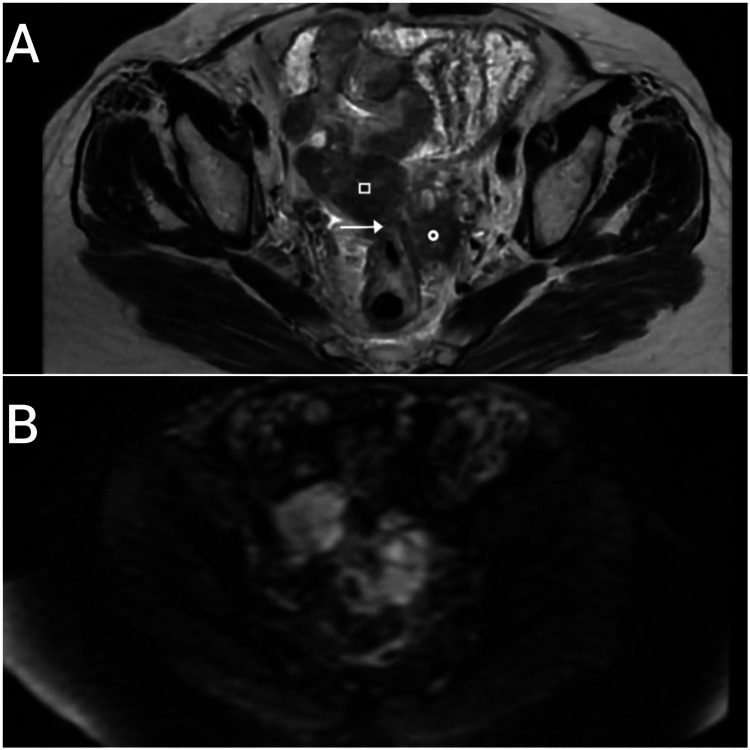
Axial T2 and diffusion of pelvic magnetic resonance imaging (MRI) (A) Axial T2 pelvic MRI: rectosigmoidien invasion (arrow), mass in the right ovary (square), mass in the left ovary (circle). (B) Diffusion pelvic MRI: diffusion restriction in both ovarian masses.
